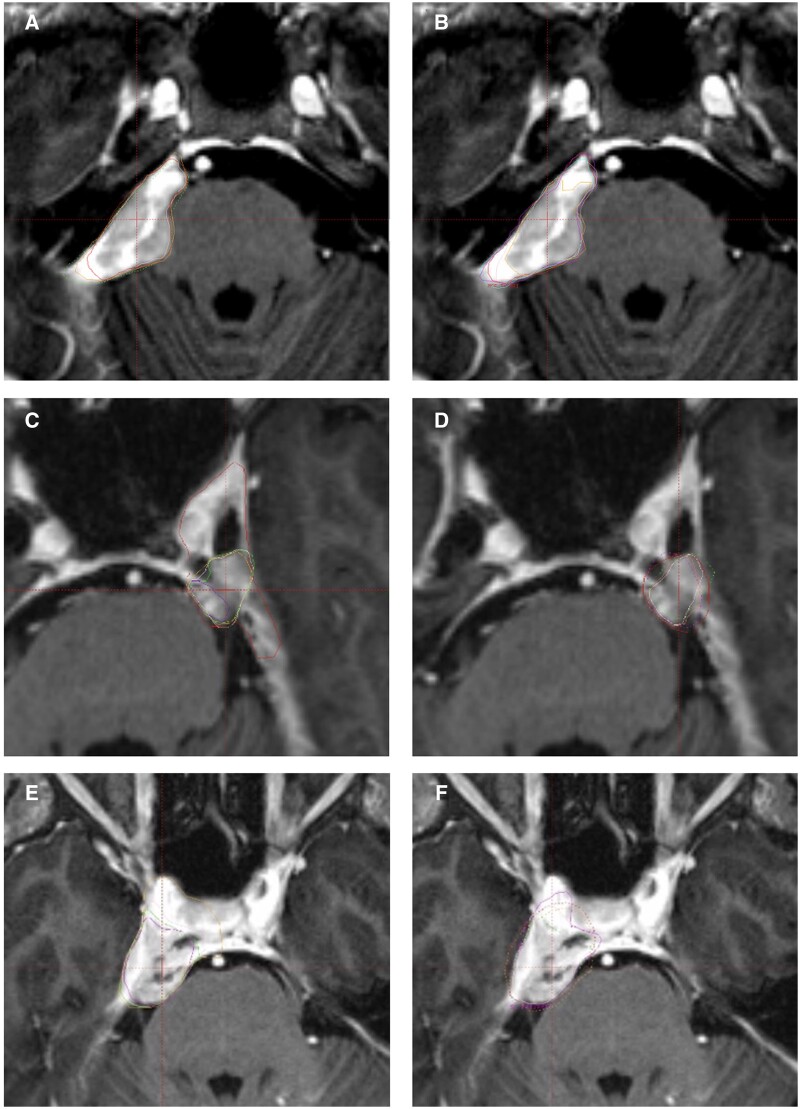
Cerebellopontine angle tumour GTV volumes comparing MRI (A) with PET/MRI (B). Cavernous sinus tumour volumes comparing MRI (C, E) and PET/MR (D, F).
